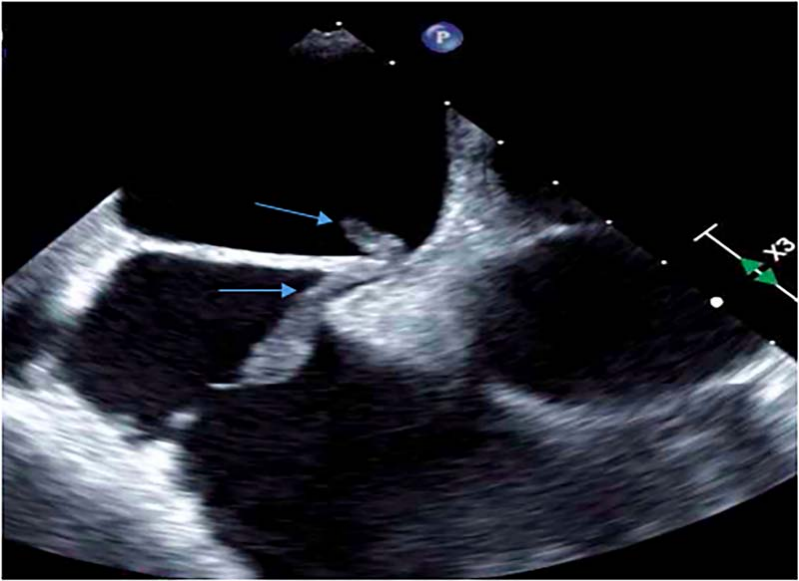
Transesophageal echocardiogram confirmed a PFO with a large thrombus seen in transit from the right atrium to left atrium.
